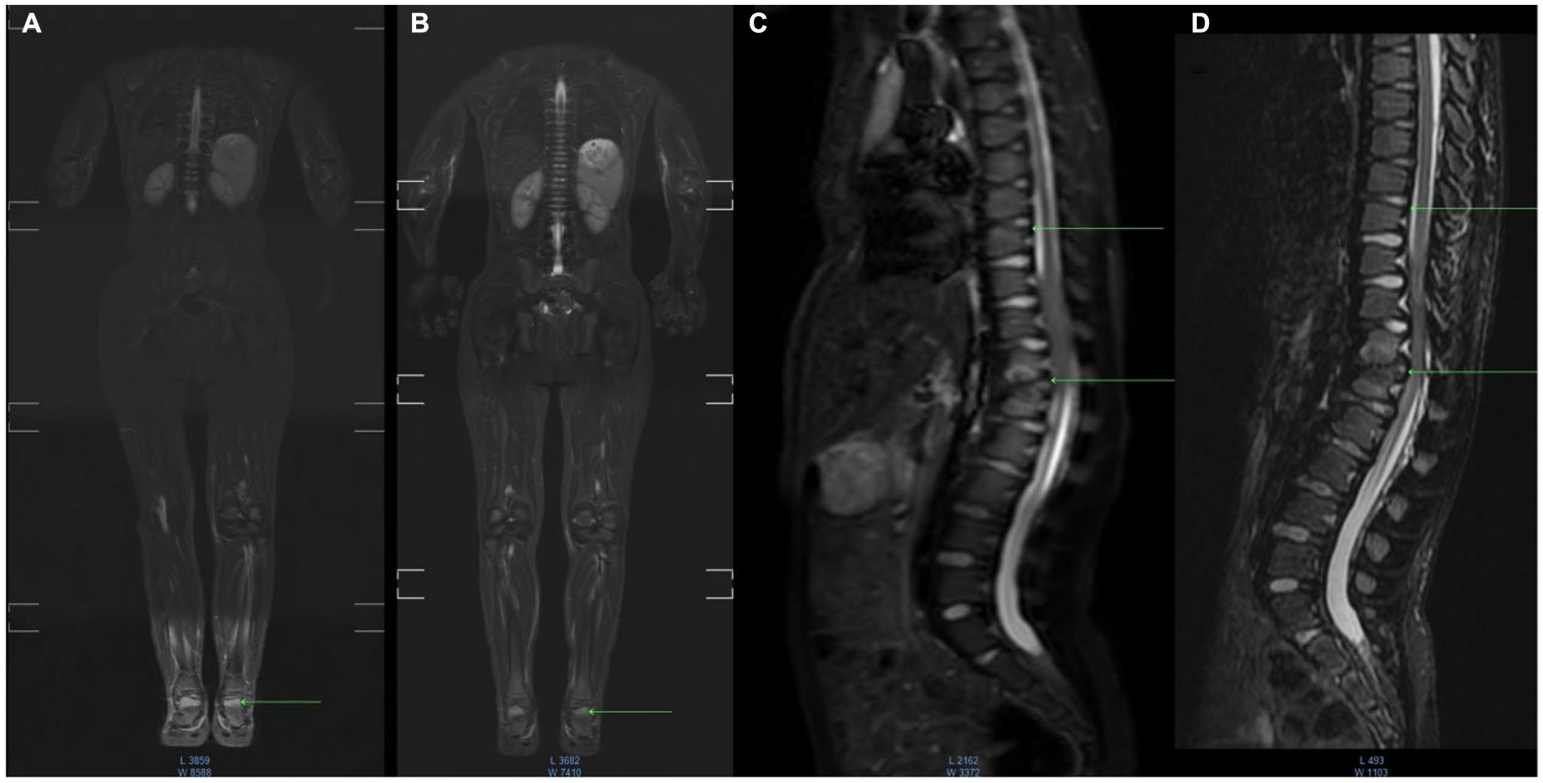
MR imaging dynamic research with fast spin-echo (SE) short inversion time inversion recovery STIR - fat suppression. Baseline or Inititial or Pre-etenercept treatment (A,C); 12 months etanercept treatment (B,D) coronal whole body, sagittal images of the spine region. Image (A) shows swelling of the bones of the foot, in the dynamics of the next study (B) the intensity of edema decreased, and destruction in the foot was not detected. In the image of the thoracic spine in the upper vertebrae, there is swelling of the bone marrow (C), whereas mechanisms of edema are not visualized (D) and the structure of the bone is not changed. In the lower Th12-L1 vertebrae, there is edema and areas of destruction of the vertebral bodies (C), and mechanisms of edema showed that the intensity of edema decreased (D) and there were destruction zones without dynamics.
